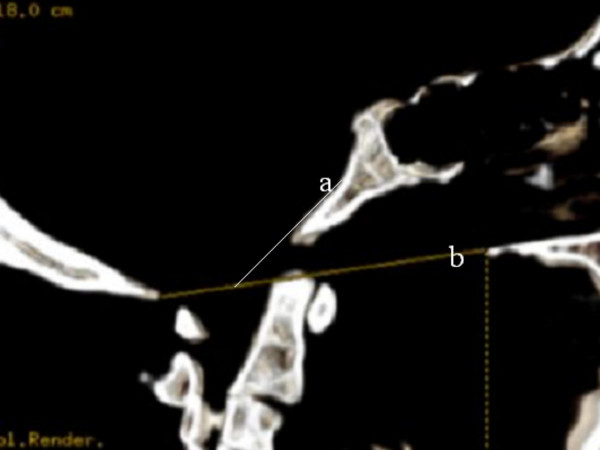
Sagittal CT image reveals anterior displacement of the odontoid process (O) to the posterior aspect of the anterior arch of C1. Note the Wachenheim clivus line* (a), which is drawn along the posterior aspect of the clivus toward the odontoid process-in our patient – the line does not intersect and is not tangential to the odontoid process-this confirms the abnormality and indicates the existence of occipito-atlas subluxation. The Chamberlain's line (b) showed no associated basilar invagination.
